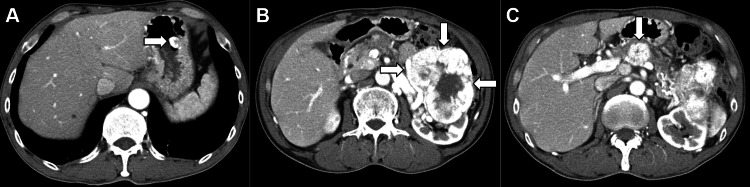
CT findings supporting the diagnosis of metastatic clear cell RCC. Abdominal CT reveals a protruded lesion in the stomach with clear enhancement (A, arrow). A primary tumor in the left kidney with heterogeneous enhancement (B, arrows). Metastatic lesions in the pancreas (C, arrow). CT, computed tomography; RCC, renal cell carcinoma
